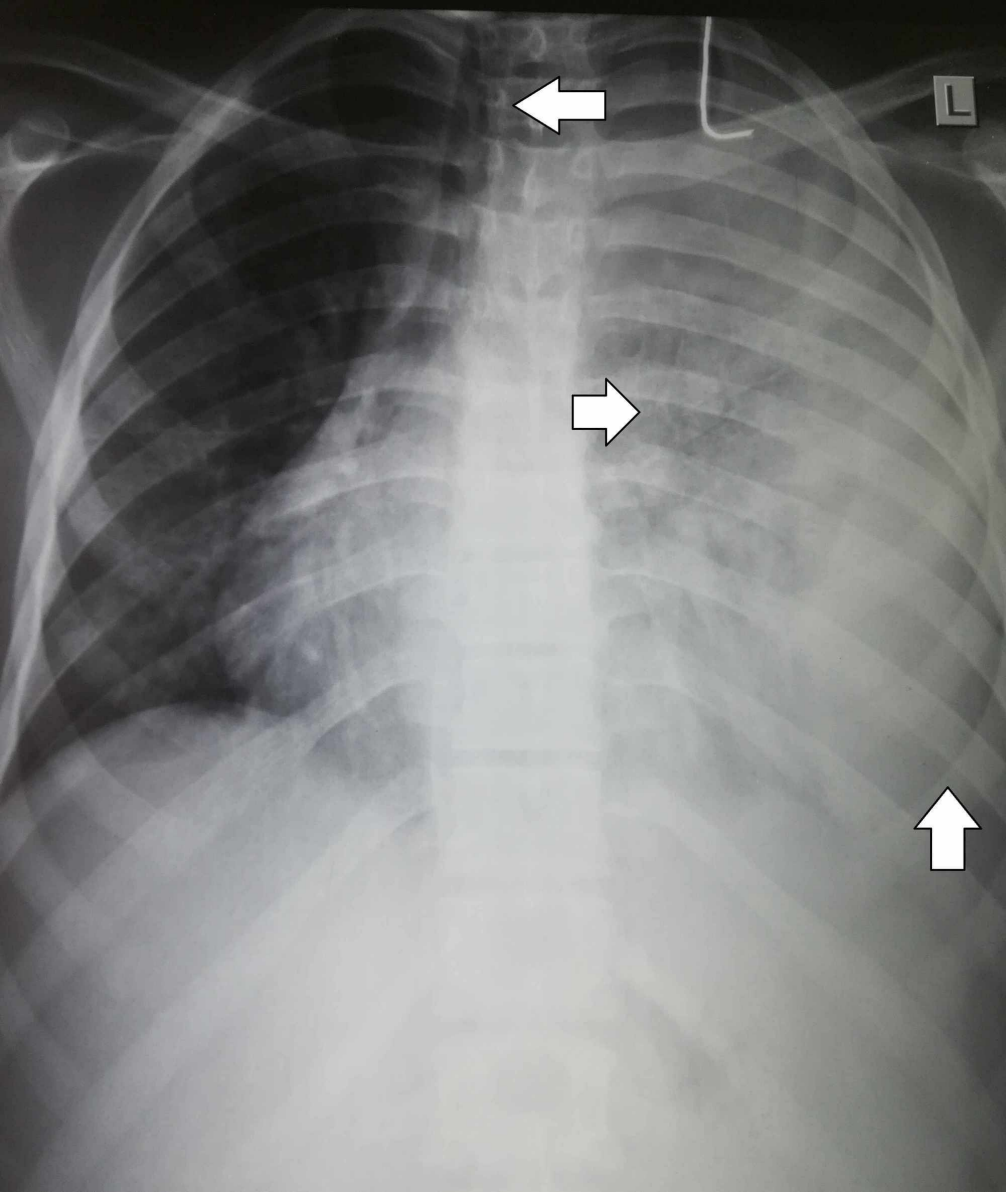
Chest radiograph showing left-sided pleural effusion with mild tracheal deviation to the right and prominent lung markings.
